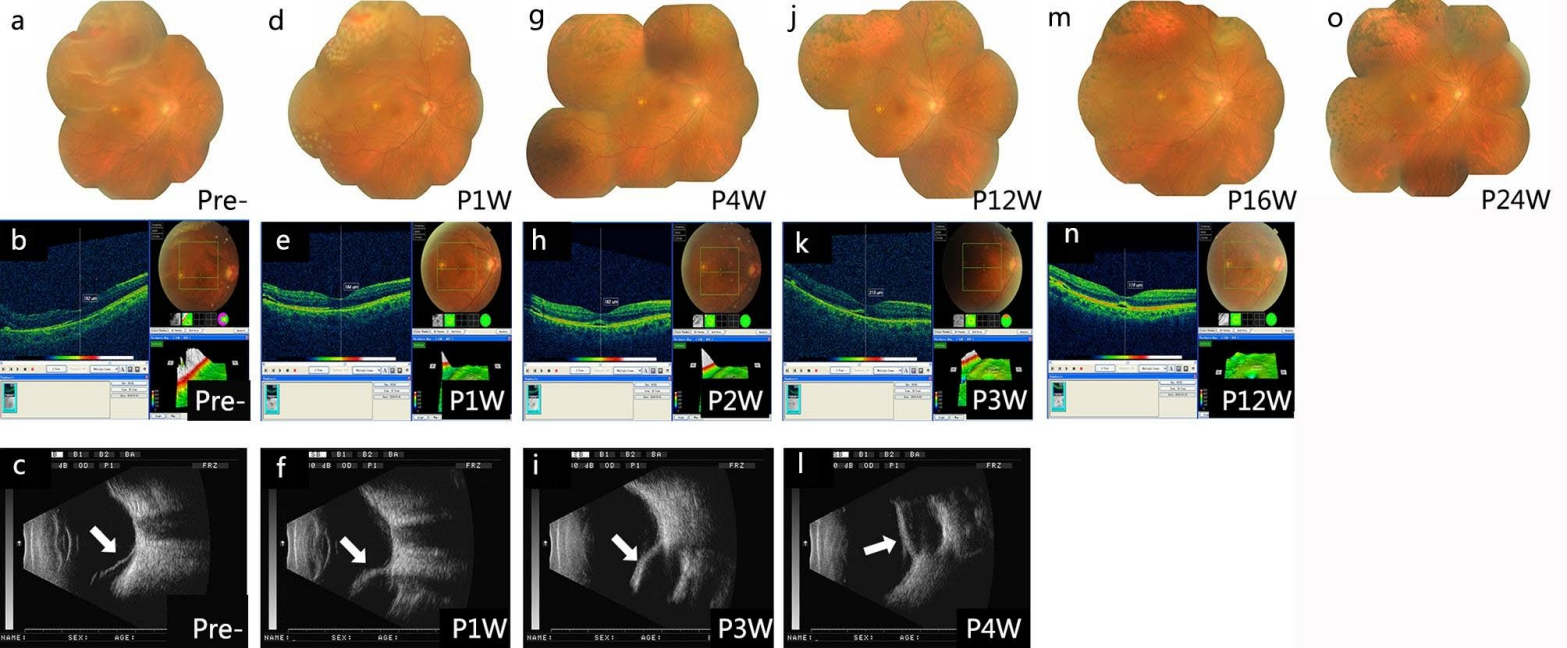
FCB scleral buckling efficacy in patient 5 (a) Fundus image showing retinal detachment pre-surgery. (b) The amplified circle area from A showing the proliferation cord (white arrow). (c) Pre-surgery B-ultrasound scan showing retinal detachment (white arrow). d–e. OCT shows an affected and detached macula. f. Fundus image of FCB scleral buckling at 1-week post-operation (P1W). g. Fundus image showing indistinct photocoagulation spots. h. Post-operative B-ultrasound scan results showing the FCB’s apparent pressure on the sclera (white arrow). i. OCT shows the much improved macular and a small amount of retained subretinal fluid. j. Fundus image at 24 weeks post-operation (P24W) showing the well-reattached retina
